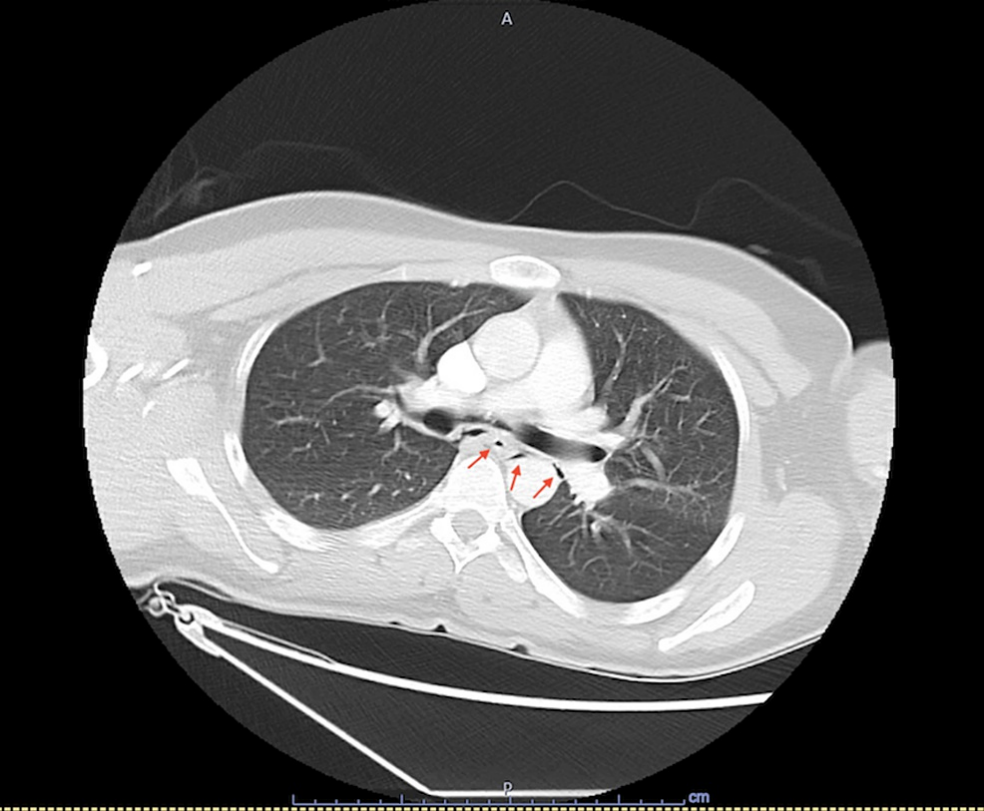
Transverse view of CT abdomen Collections of air surrounding the esophagus (red arrows)
